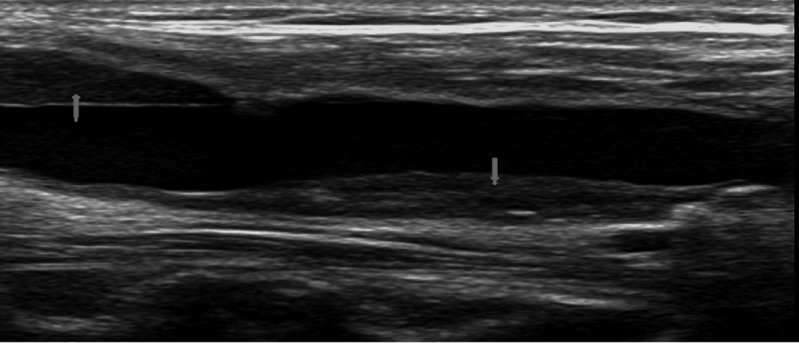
Carotid ultrasound showing echolucent plaques. Plaque in the far wall of the common carotid artery (arrow pointing down) and in the near wall at the carotid bifurcation (arrow pointing up).
